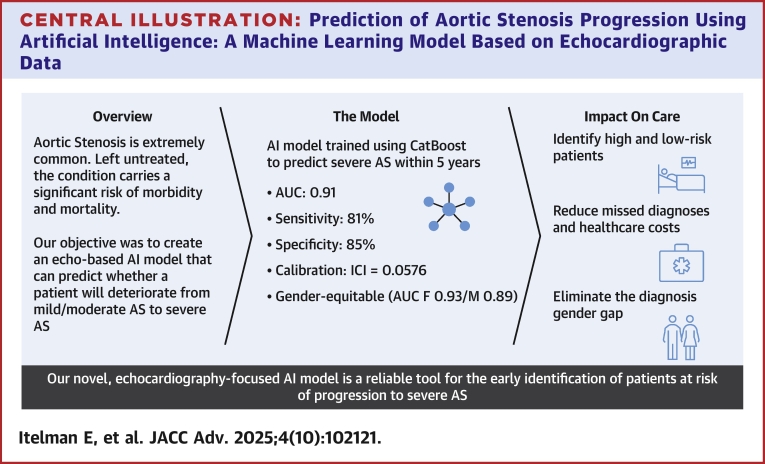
Prediction of Aortic Stenosis Progression Using Artificial Intelligence: A Machine Learning Model Based on Echocardiographic Data
